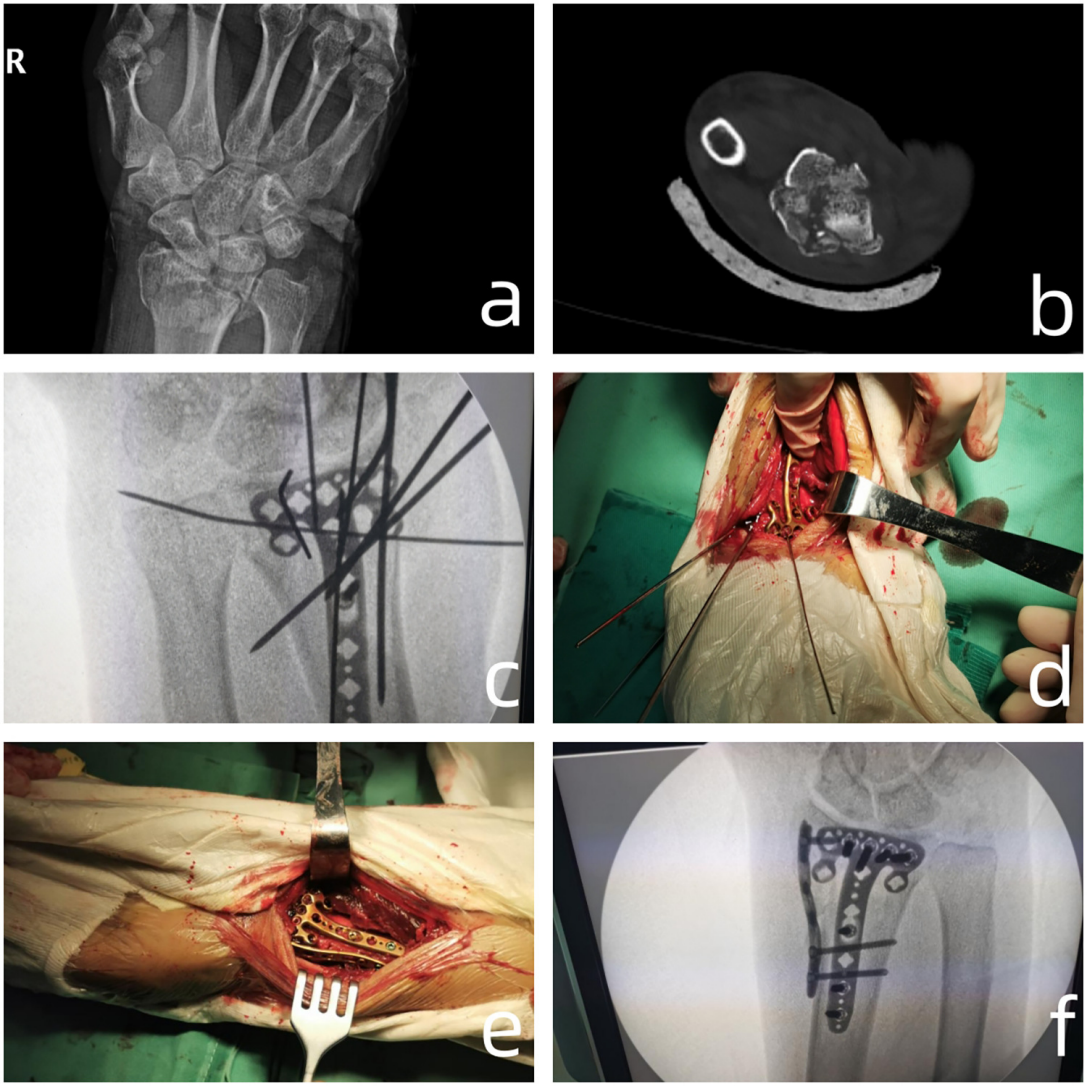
(a,b) The articular surface fracture as shown by x-ray and CT axial scan; (c,d) placement of the plate after temporary fixation with kirschner wires revealed poor reduction of the radial styloid process, leading to placement of a radial plate; (e,f) surgical and radiographic outcomes of dual-plate fixatio.
